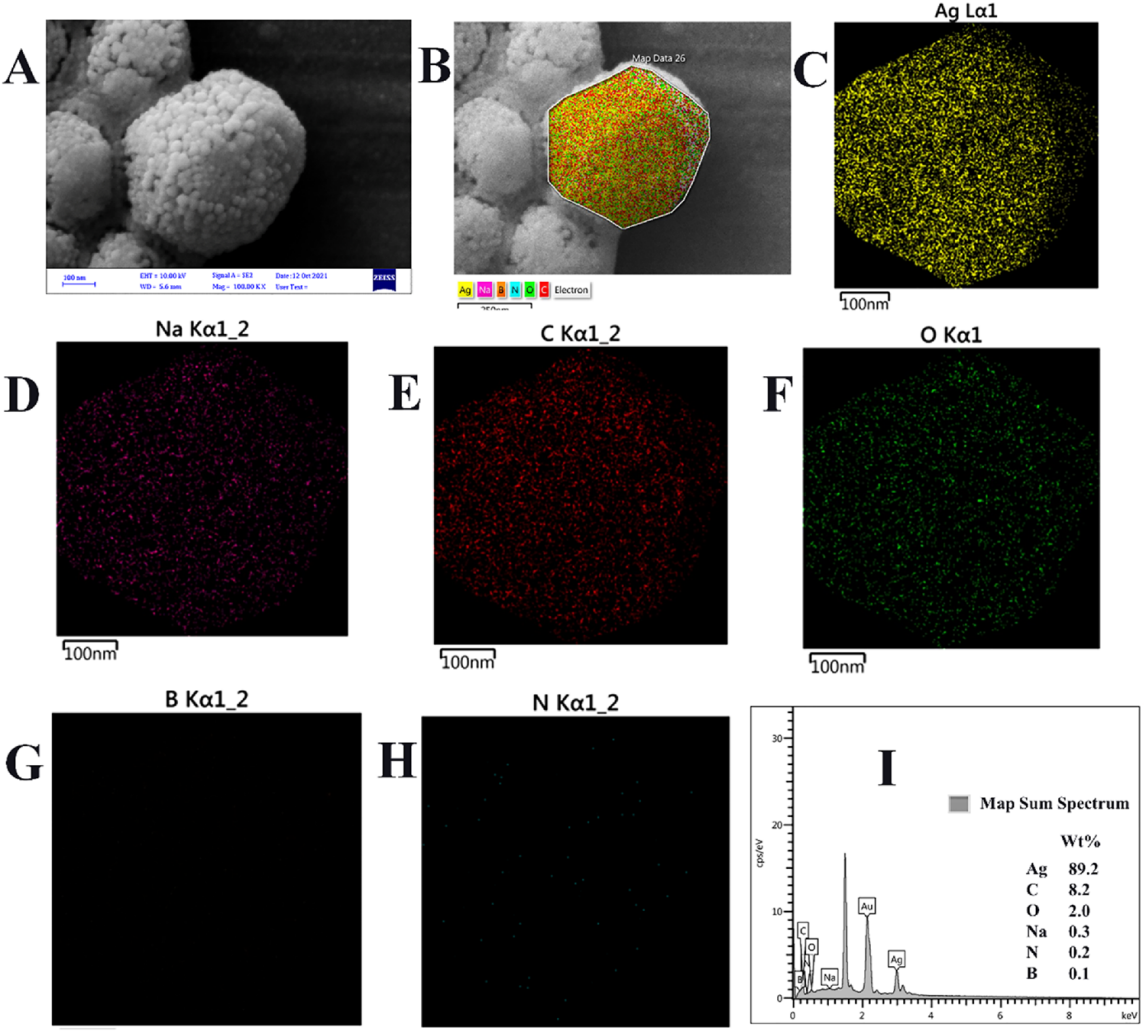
SEM image (A); elemental X-ray mapping (from B to H) and EDX analysis (I) of the area marked with a multifaceted (in B); of the as-prepared Ag NR@PEI-Ag nanohybrid.
